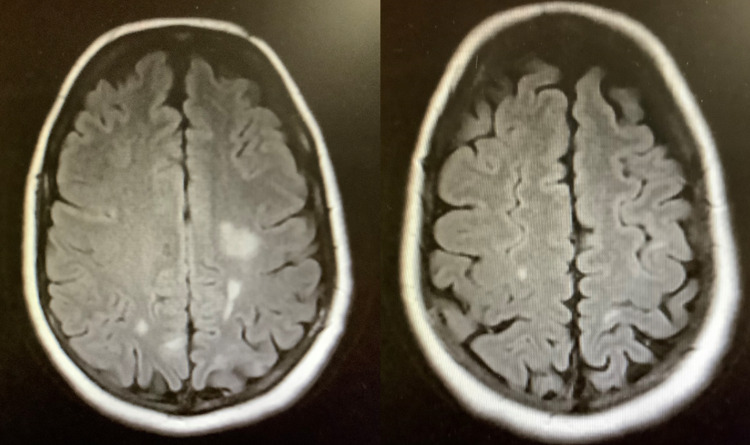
Brain MRI demonstrating subcortical hyperintense lesions of the bilateral parietal lobes consistent with demyelination
